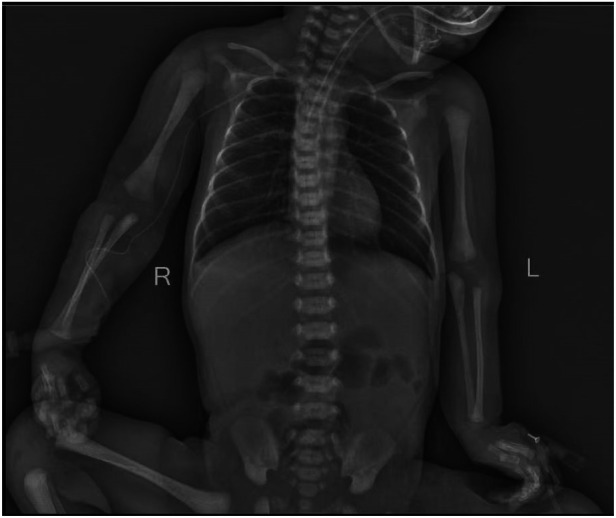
The x-ray chest examination results of the proband.
